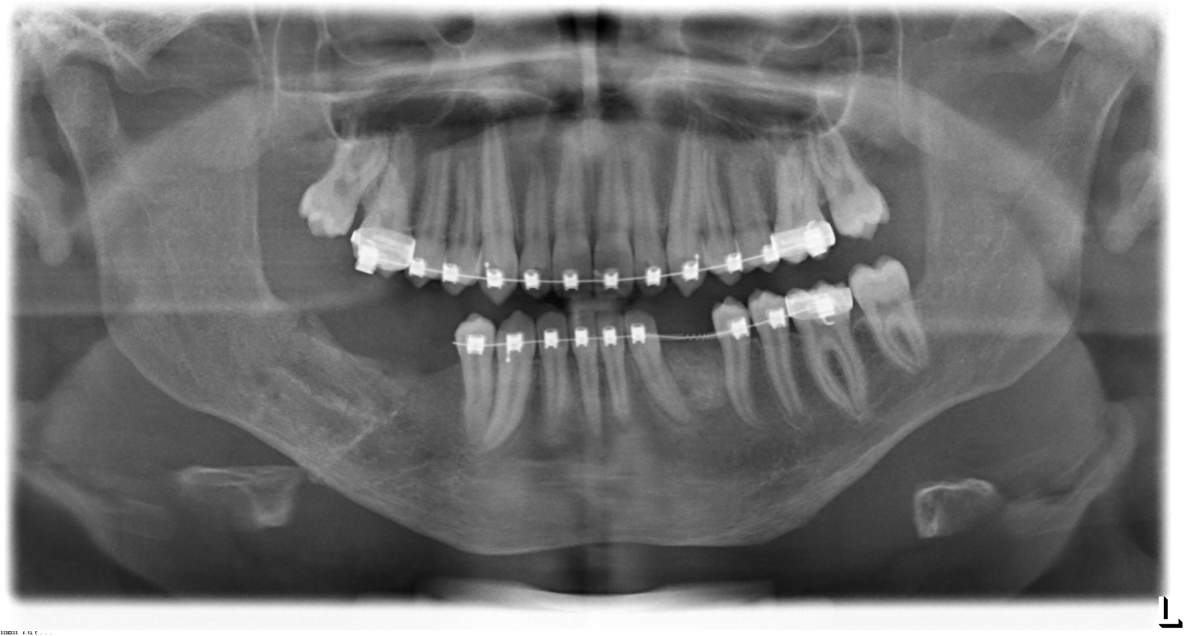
Orthopantomogram after finalization of the consolidation phase. A sufficient osteogenesis in the distracted area is achieved
